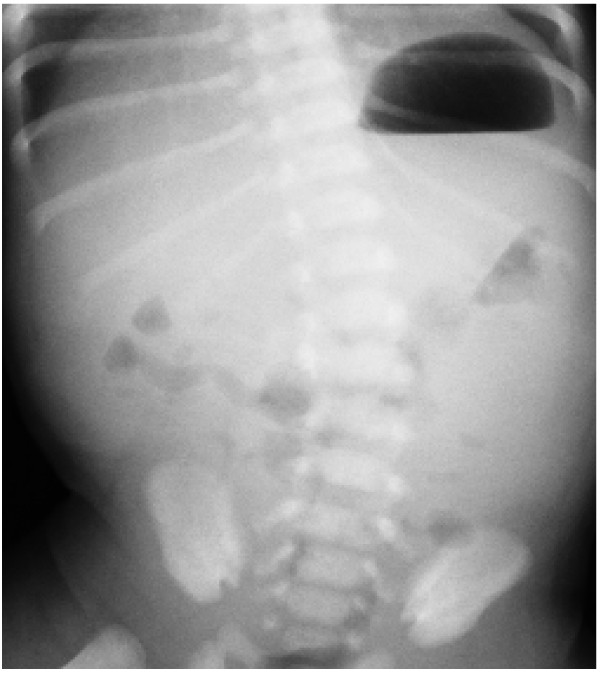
A plain abdominal radiograph shows a paucity of gas in the distal bowel.
